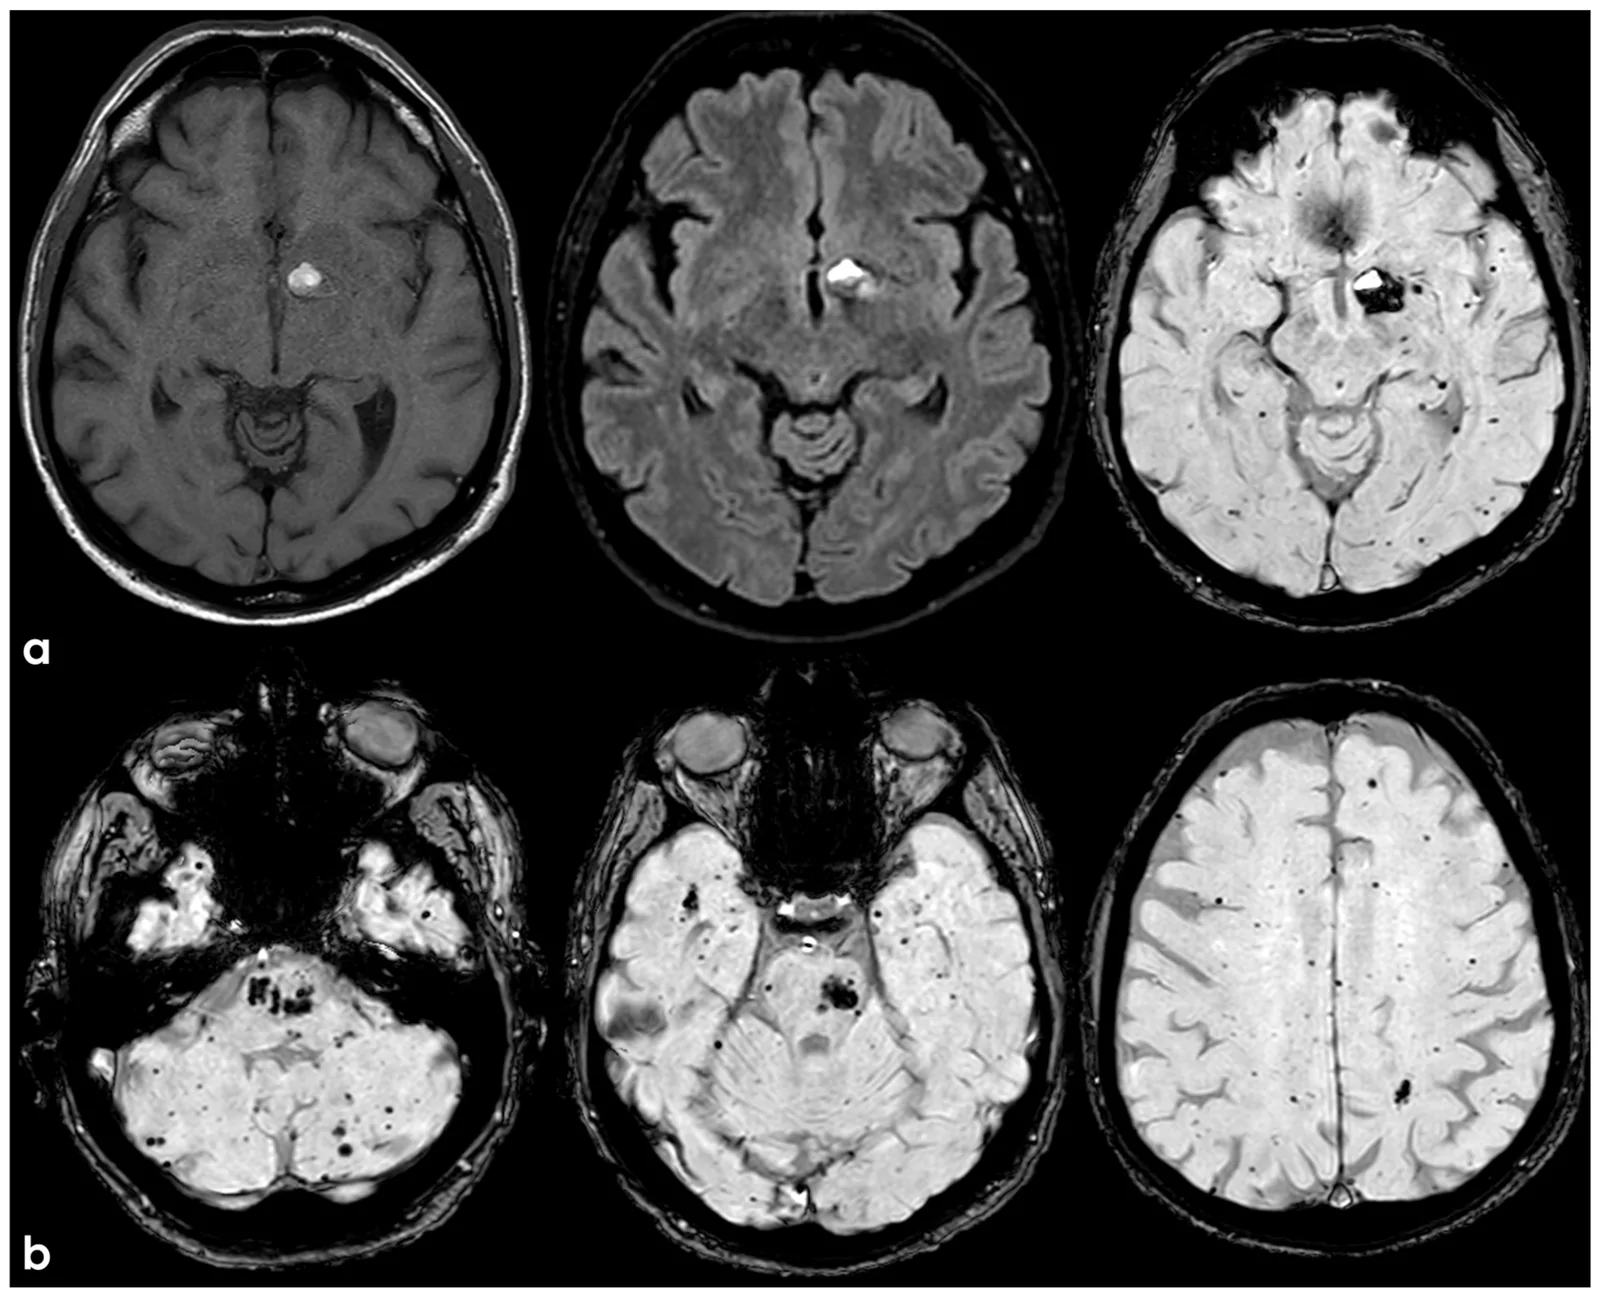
Brain MRI of a 74 yo man with a focal seizure. (Panel a): axial T2, FLAIR and SWI, showing a left deep anterior diencephalic lesion consistent with subacute bleeding of a cavernous angioma. (Panel b): Other axial SWI slices showing multiple hypointense lesions confirmed as CCMs. The patient underwent genetic testing, finding a known pathogenic mutation of *CCM1* gene.
